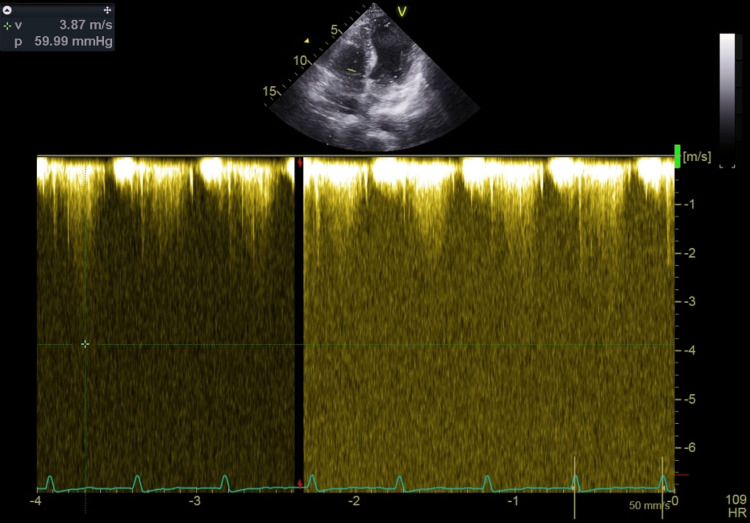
Continuous wave TTE at the LVOT obstruction and mitral valve showing a gradient of 59 mmHg after initiation of bisoprolol TTE: transthoracic echocardiography, LVOT: left ventricular outflow tract
